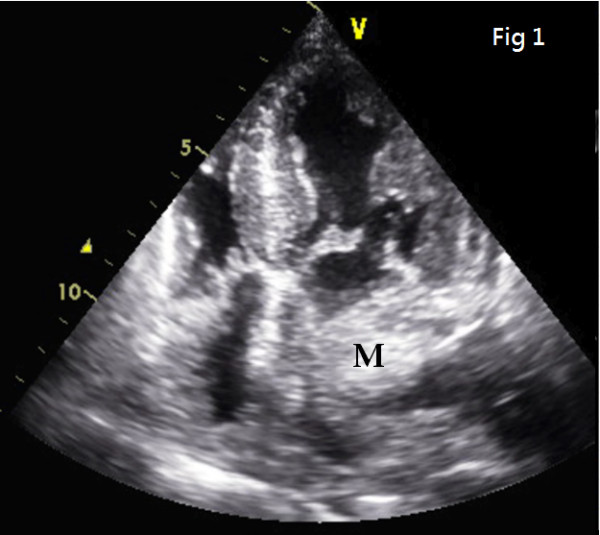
Two-dimensional echocardiography view: a heterogeneous mass as large as 2.3 × 2.4 cm occupying the left atrium (LA) is revealed.
